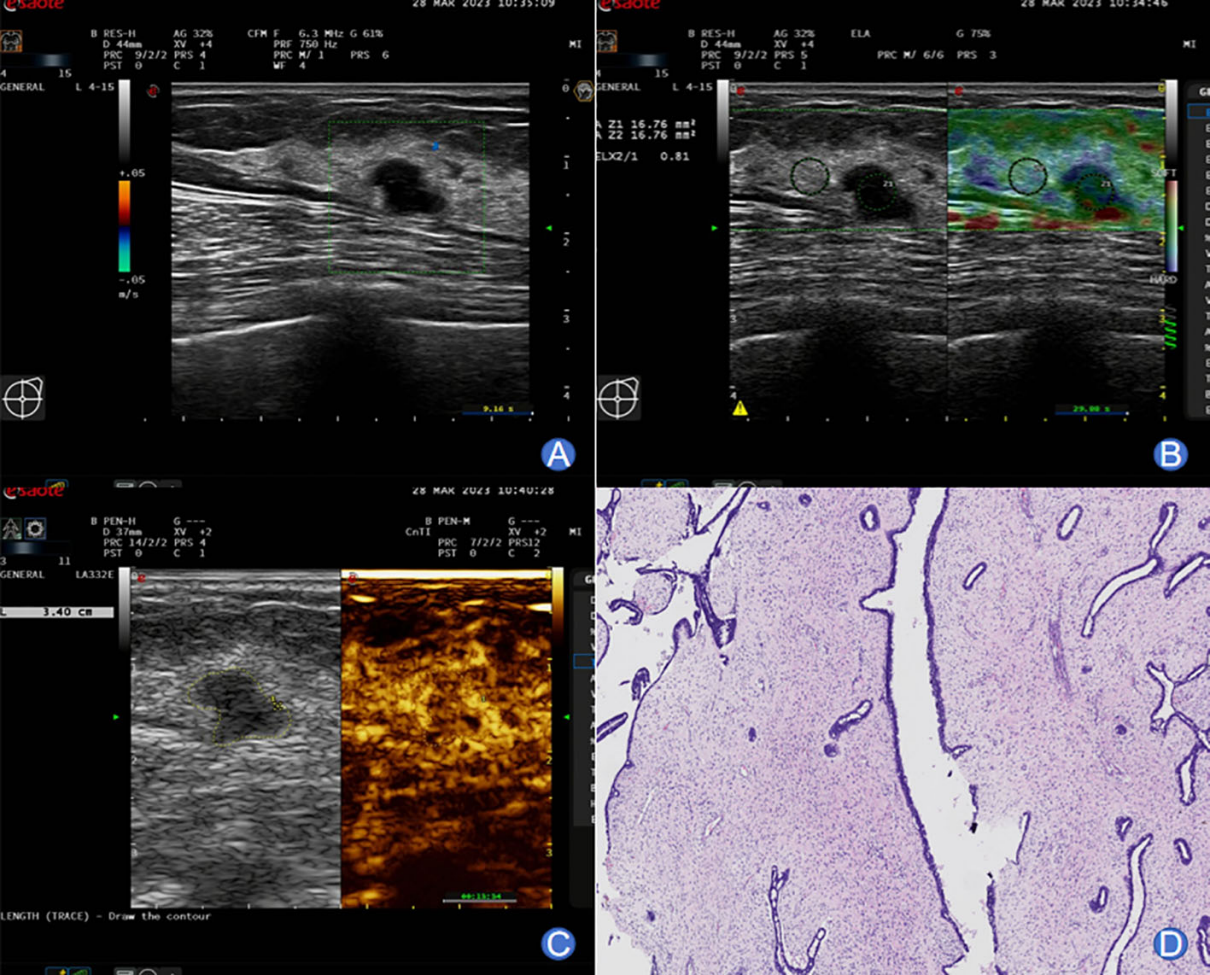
A 49-year-old female with typical images of benign breast tumor. (A) Conventional ultrasound imaging unveiled a well-defined, approximately 11×8 mm hypoechoic nodule in the left breast. The lesion exhibited irregular morphology with an aspect ratio greater than 1. Echoes remained homogeneous, and no noticeable blood flow signals were observed. (B) SWE imaging displayed a relatively soft nodular texture, with an Emax value of 45 kPa. (C) Following a bolus injection of 4.8 mL contrast agent through the superficial vein at the elbow, enhancement was monitored at 7 seconds, preceding the enhancement in surrounding glandular tissues. Peak enhancement was attained at 14 seconds, demonstrating slightly lower-level and homogeneous enhancement. The lesion exhibited a clear boundary and a surrounding ring-like enhancement pattern. Post-enhancement, the lesion’s size remained unchanged, and washout-type alterations occurred earlier than in the surrounding glandular tissues. (D) Pathological examination yielded a diagnosis of fibroadenoma of the breast.
